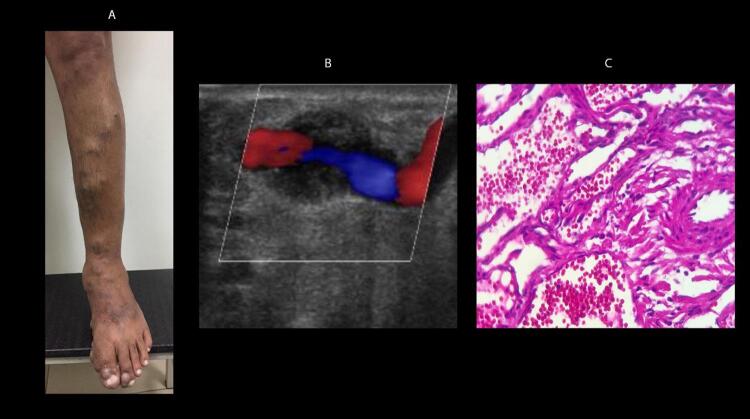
Hemangioendotelioma venoso. A: aspecto clínico; B: imagem hipoecogênica circundando uma veia superficial à ultrassonografia com mapeamento de fluxo a cores; C: exame anatomopatológico.
